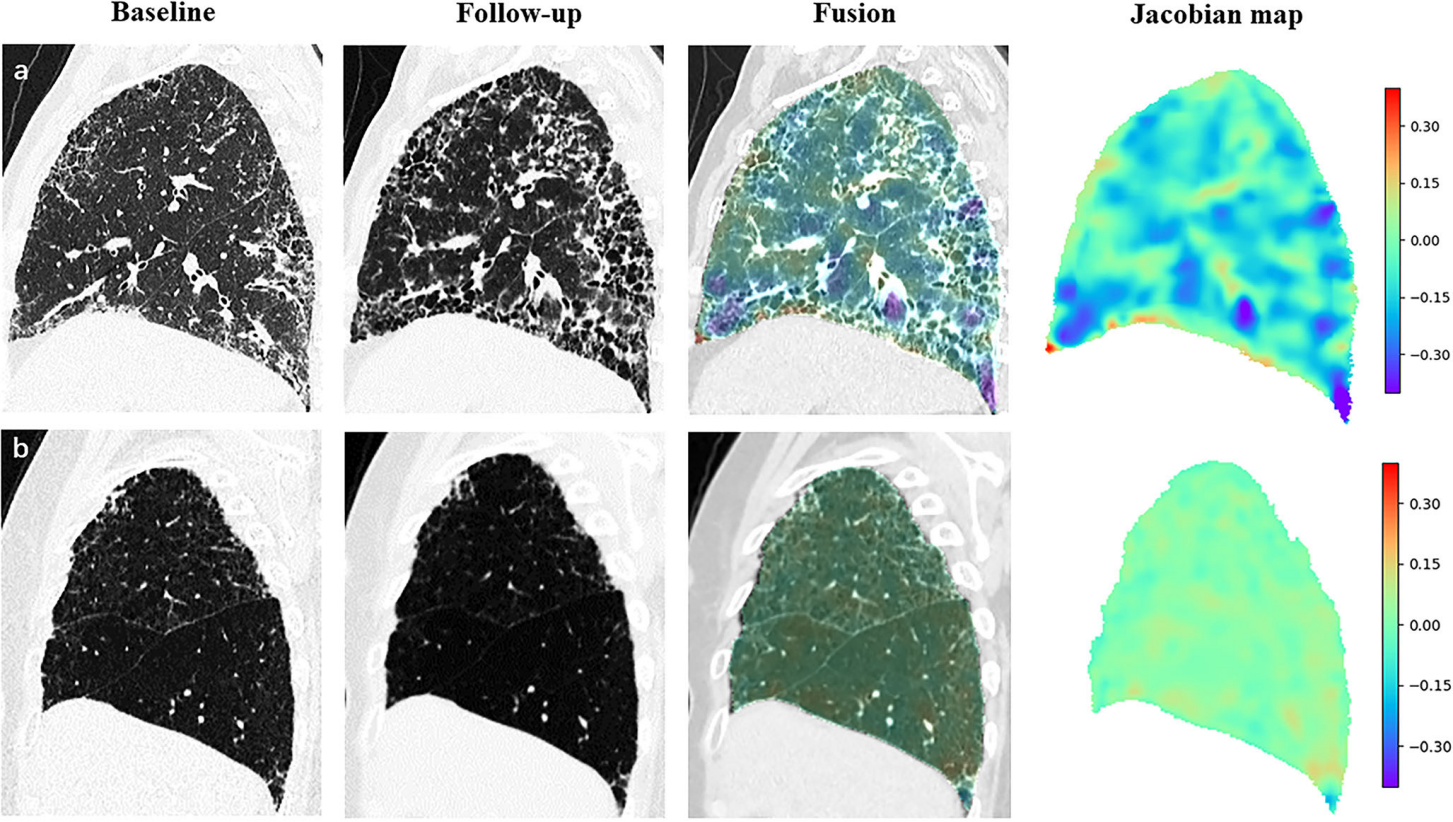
The representative Jacobian maps in two patients with IPF. a A 63-year-old patient with a follow-up interval of 30 months. Follow-up CT showed a significant increase in honeycombing and reticulations compared to baseline CT. Both visual and functional assessments supported disease progression. A Jacobian map showed diffuse lung shrinkage in correspondence with areas of increased fibrotic lesions and with a negative mean log_jac value. b A 77-year-old patient with 7-month follow-up interval. Follow-up CT showed no significant change in the lesions compared to baseline CT. Both visual and functional assessments indicated disease stability and the mean log_jac value was 0
